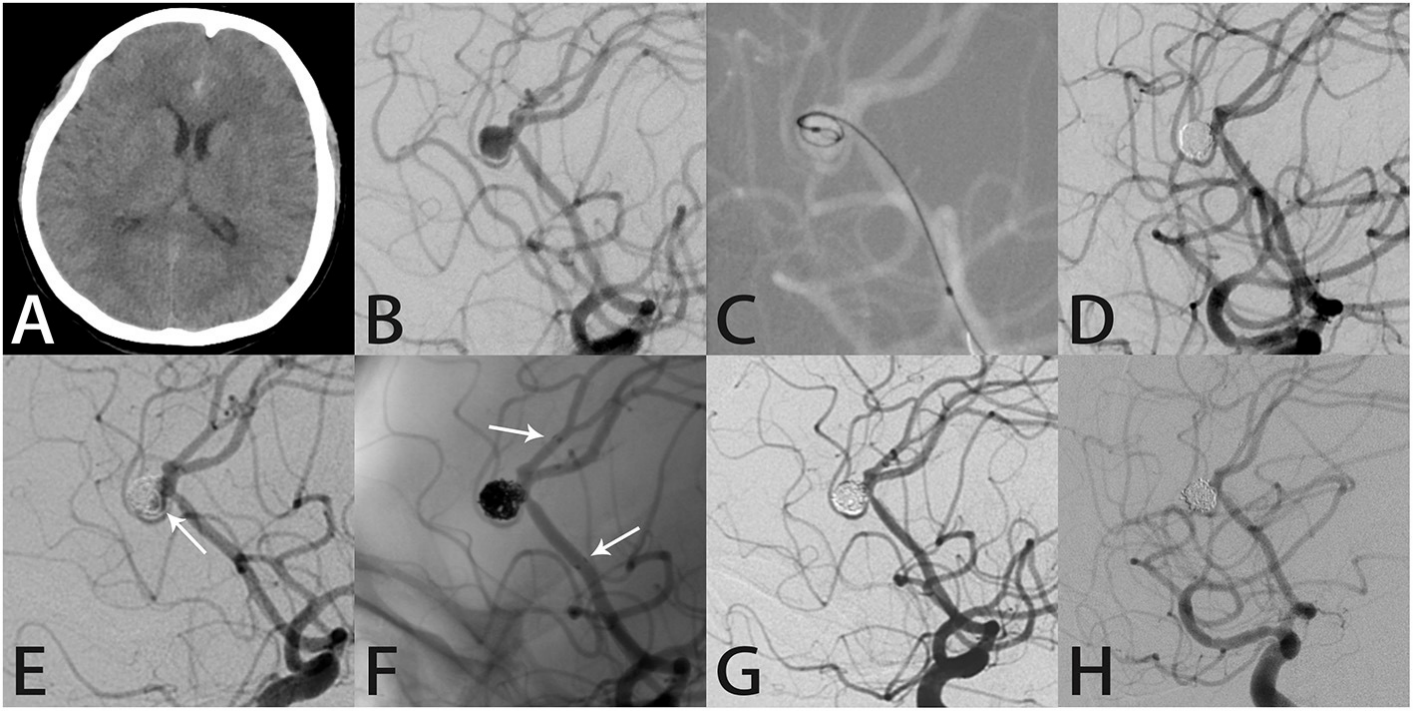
Images from a 48-year-old woman with a right pericallosal aneurysm (case 4). (A) Computed tomography showed subarachnoid hemorrhage in the longitudinal fissure. (B) Angiography showed a right pericallosal aneurysm. (C) During treatment, an Echelon 10 microcatheter (Medtronic, Dublin, Ireland) was delivered into the aneurysm sac to place the coils. (D) The aneurysm was occluded completely after coil embolization. (E) Follow-up angiography 11 months later showed recurrence in the aneurysm neck (white arrow). (F) Intraprocedural angiography showed the deployed Atlas stent (3.0 × 21 mm) covering the aneurysmal neck and coils densely packed within the sac (white arrows indicate the ends of the stent). (G) Angiography immediately after the procedure showed the aneurysm was occluded completely. (H) Follow-up angiography 7 months later showed complete aneurysmal occlusion with the coils densely packed within the aneurysm.
